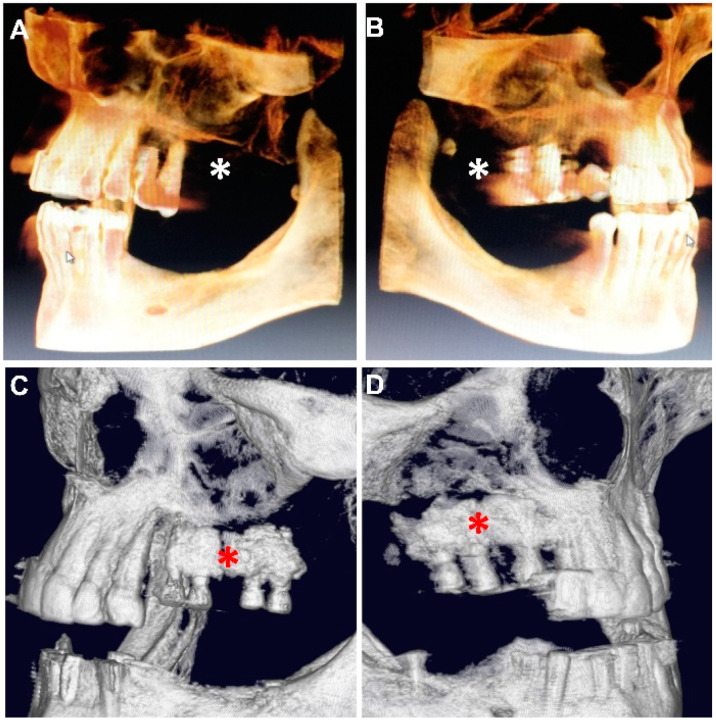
Bone loss and Paste-CPC-filled defect were visualized using 3D reconstruction resulting from CBCT imaging. The large defect area (white asterisks) resulting from significant loss is of the buccal bone on both sides. Bone substitute material surrounds the new implants after injection (red asterisks) using the window approach ((A,C): left side, (B,D): right side).
